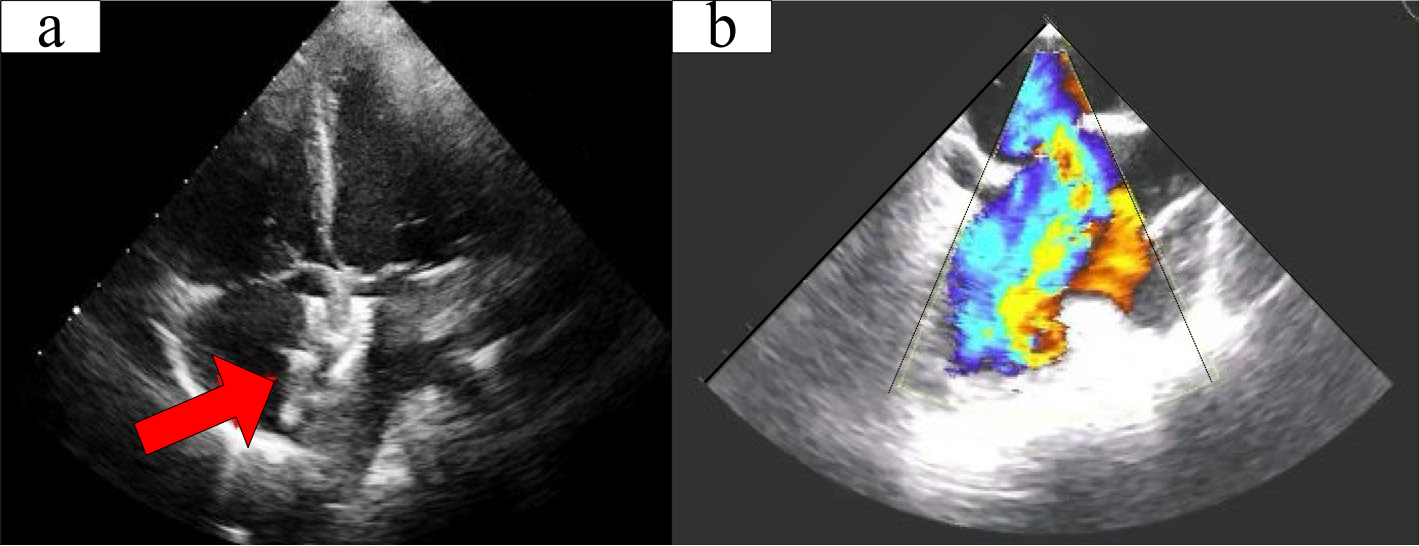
Comparison between two-dimensional ultrasound and three-dimensional echocardiography
